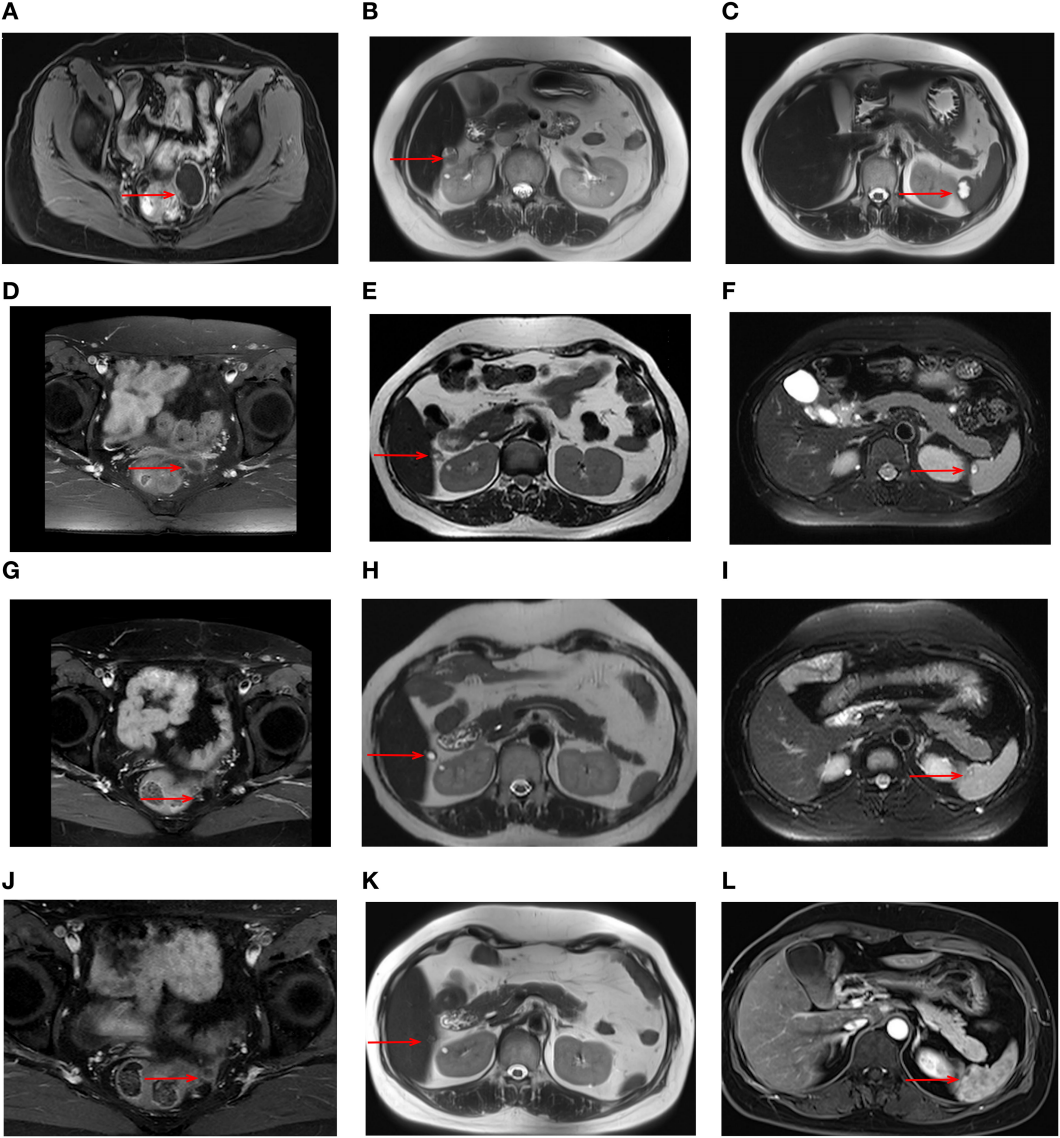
MRI images during treatment with the triple regimen. MRI images of the tumors (red arrows) are shown at different stages; Prior to the treatment: pelvic mass (A) liver capsule (B) spleen (C) After 6 cycles: pelvic mass (D) liver capsule (E) spleen (F) After 22 cycles: pelvic mass (G) liver capsule (H) spleen (I) After 33 cycles: pelvic mass (J) liver capsule (K) spleen (L).
